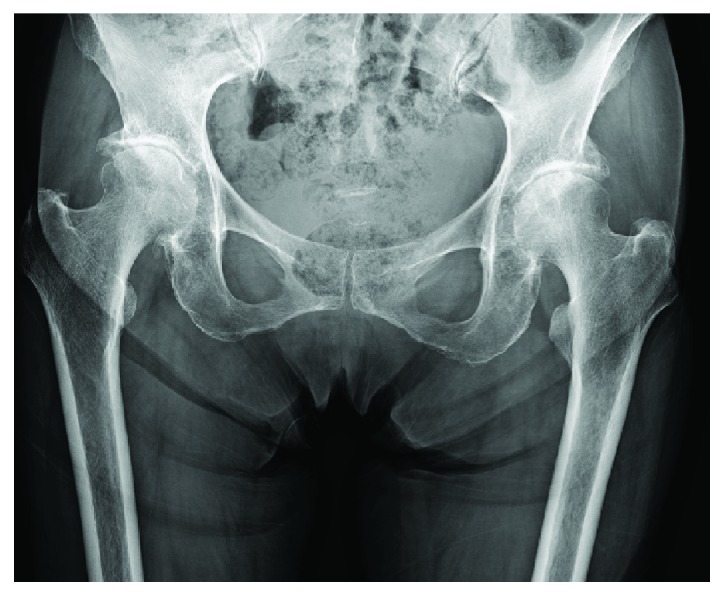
Preoperative radiograph showing osteoarthritis secondary to developmental dysplasia of the hips.
